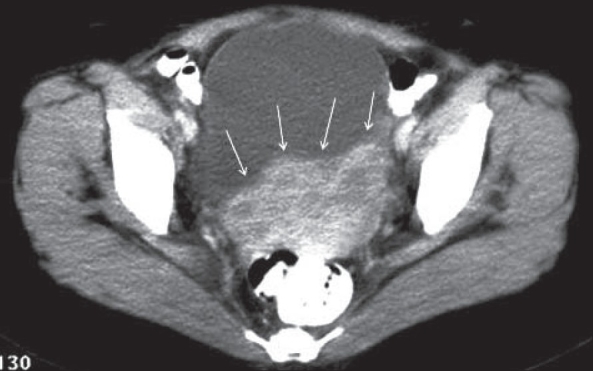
Axial CT image through the mass shows heterogeneously enhancing nodular masses (arrows)
